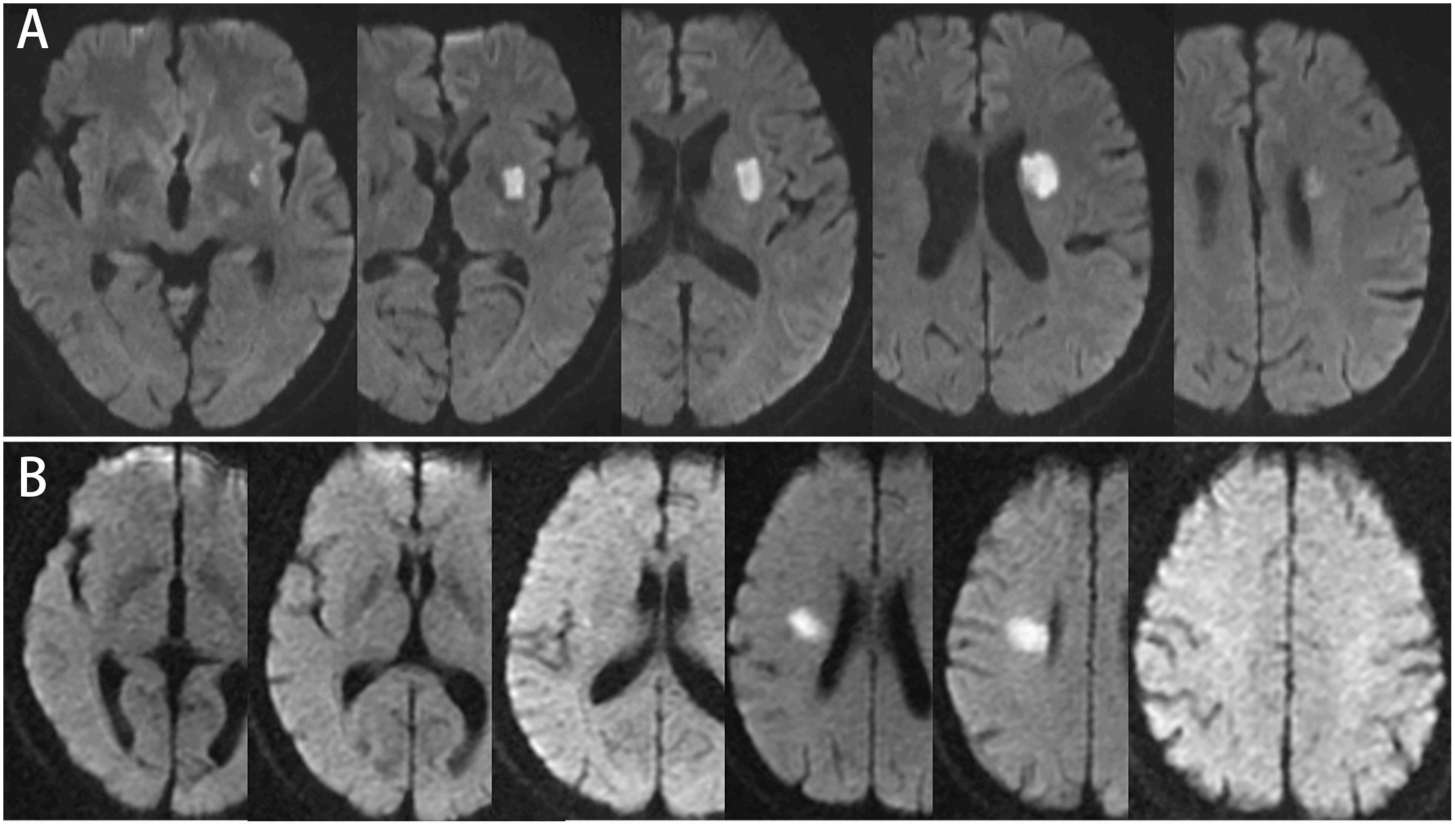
(A) 57-year-old male, consecutive slices DWI imaging: infarct lesion ≥4 slices, involving the base of basal ganglia, but the maximum axial diameter is 21.7 mm. (B) 52-year-old male, consecutive slices DWI imaging: infarction lesion ≤3 slices, involving radiation corona, and the maximum diameter of axis is 21.2 mm.
